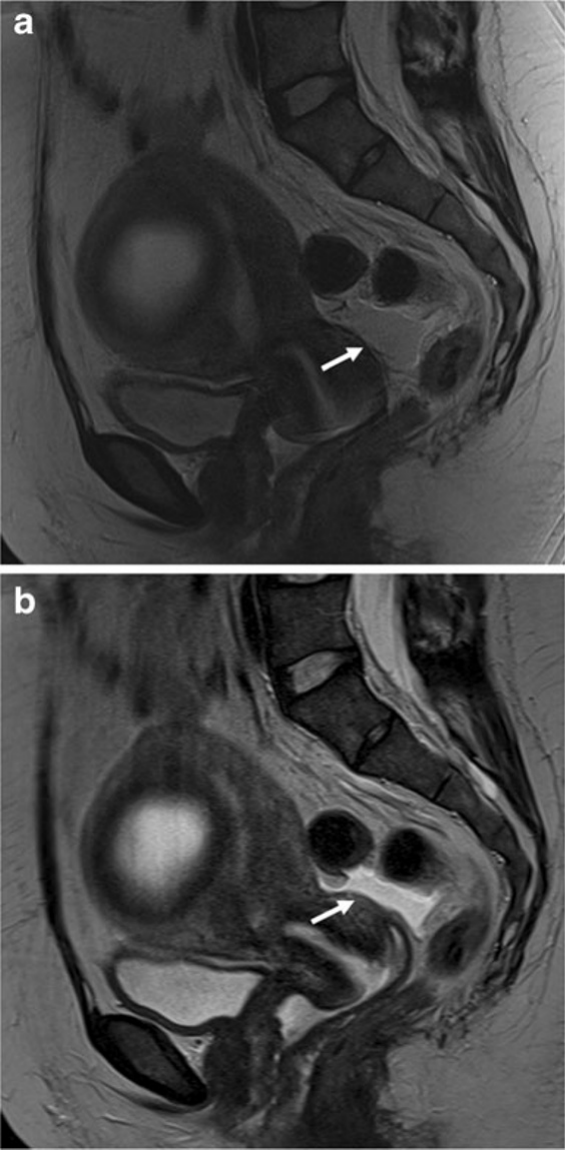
MRI in a 37-year-old patient with necrotic intramural leiomyoma. a T2-weighted BLADE sequence. b T2-weighted conventional TSE sequence. Due to the lower SNR and CNR and lower TE, the free fluid in the pouch of Douglas (*arrows*) is less obvious on the BLADE sequence. This could result in diagnostic misinterpretations
